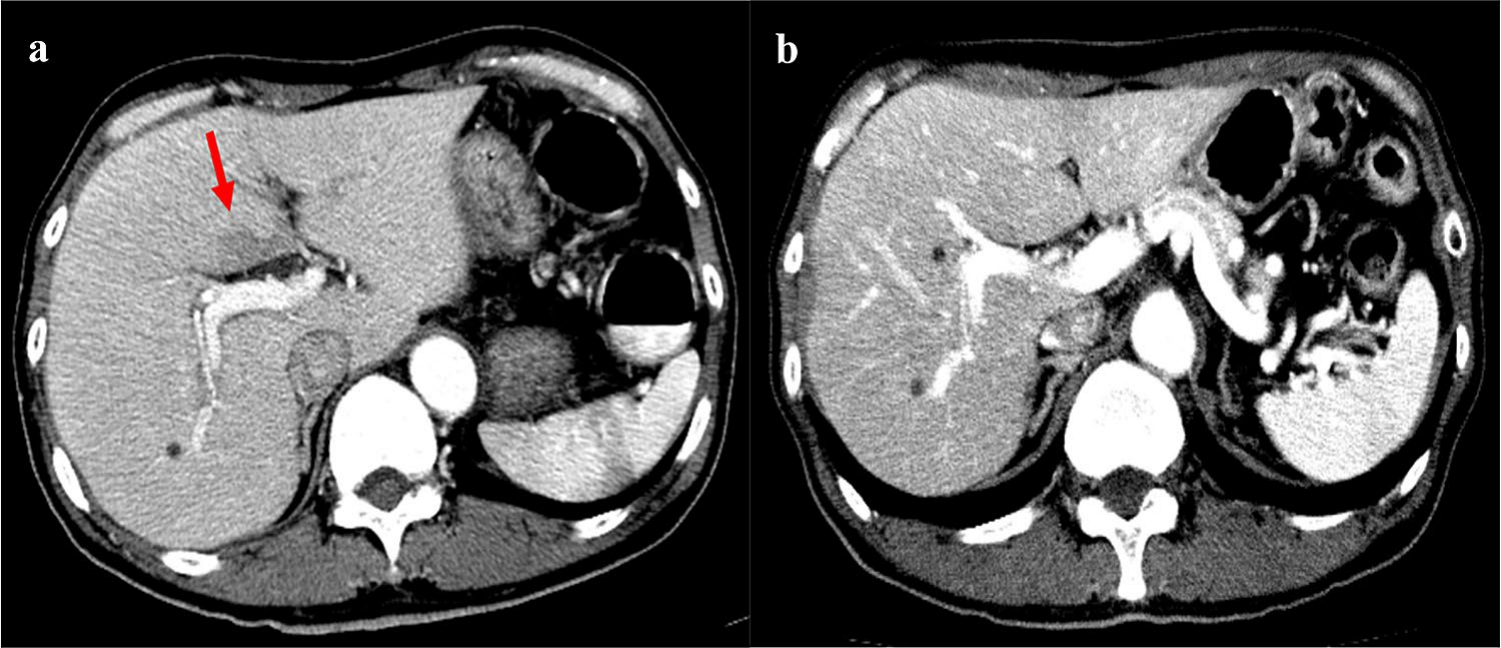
Hypovascular pseudolesion observed in a 58-year-old man. Early-phase CT (a) obtained after CTC with carbon dioxide expansion (CE-CTC) showed a hepatic pseudolesion seen as a hypo-attenuating area at the posterior edge of the left medial segment (arrow). On the early-phase CT (b) obtained without CTC procedures, a hepatic pseudolesion was not demonstrated. The contrast enhancement value of the liver parenchyma after CTC was higher than that without CTC procedures (right lobe: 66 vs. 51, left lobe: 75 vs. 67)
